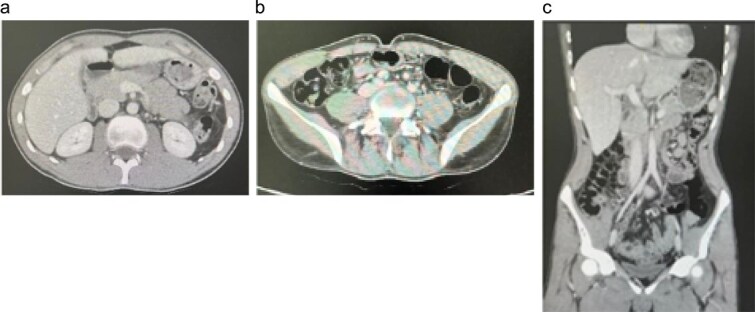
Follow-up contrast-enhanced CT abdomen in Case 1. (a) Axial image through the upper abdomen showing interval disappearance of the previously described hepatic lesions. (b) Axial image at the level of the right iliac fossa demonstrating resolution of cecal/right colonic wall thickening and surrounding inflammatory changes. (c) Coronal reformatted image confirming normalization of right colon and liver appearance, consistent with an excellent radiologic response to prolonged voriconazole therapy.
